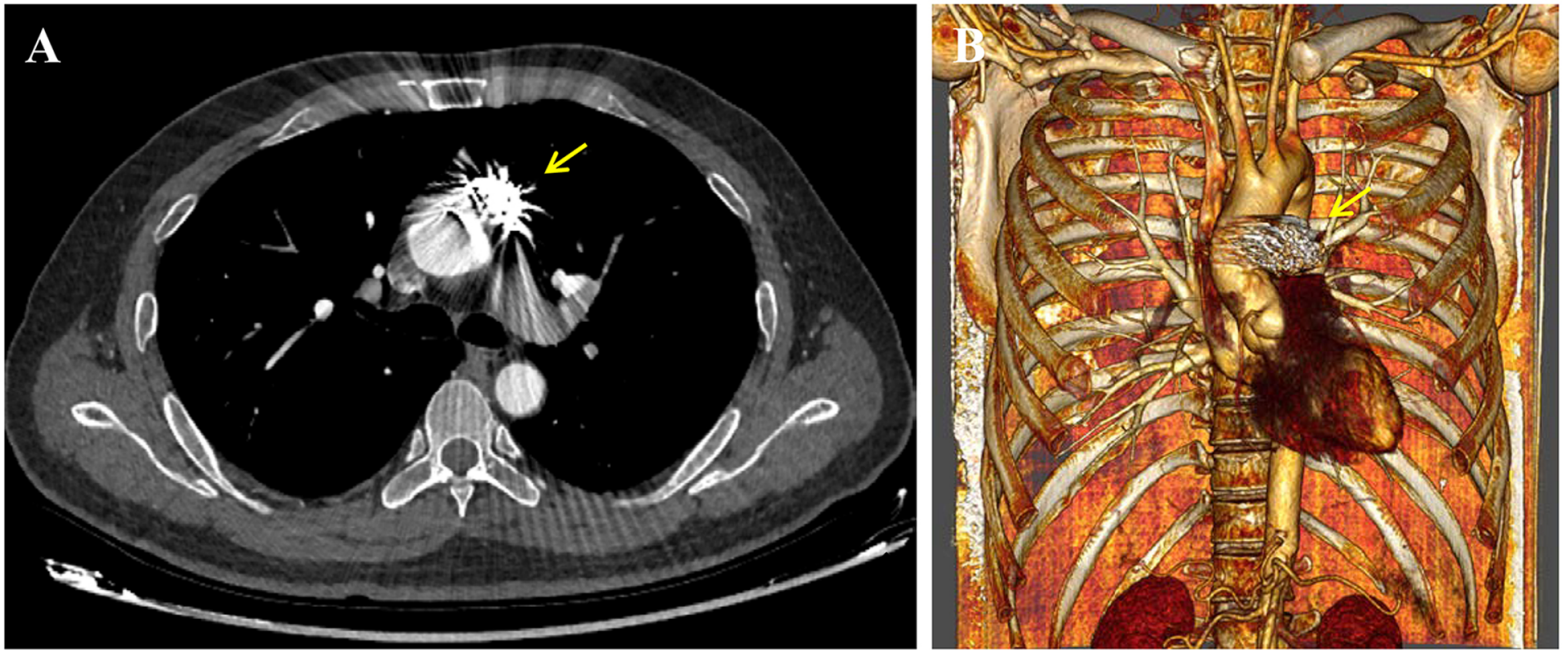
Postoperative CT angiography at the 1-year follow-up depicted (A) complete thrombosis and reduction in the size of the pseudoaneurysm sac. (B) 3D reconstructed image showed secure positioning of the coils without any evidence of migration (indicated by the yellow arrow).
